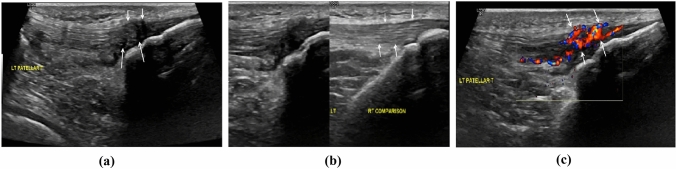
Ultrasonography images of the left patellar tendon, in a middle-aged elite athlete with persistent anterior knee pain. a Longitudinal B-mode image showing the inhomogeneous echogenicity in the distal anterior tendon in keeping with degeneration (short arrows) and microtears in the posterior aspect (long arrows). b On the right side of the image, the normal right patellar tendon is shown for comparison (arrows). c Longitudinal color Doppler image shows florid neovascularity in the degenerated foci (arrows). (Courtesy: Dr. G. Kakkos and Prof. A. Karantanas, Dpt. of Medical Imaging, Heraklion University Hospital)
